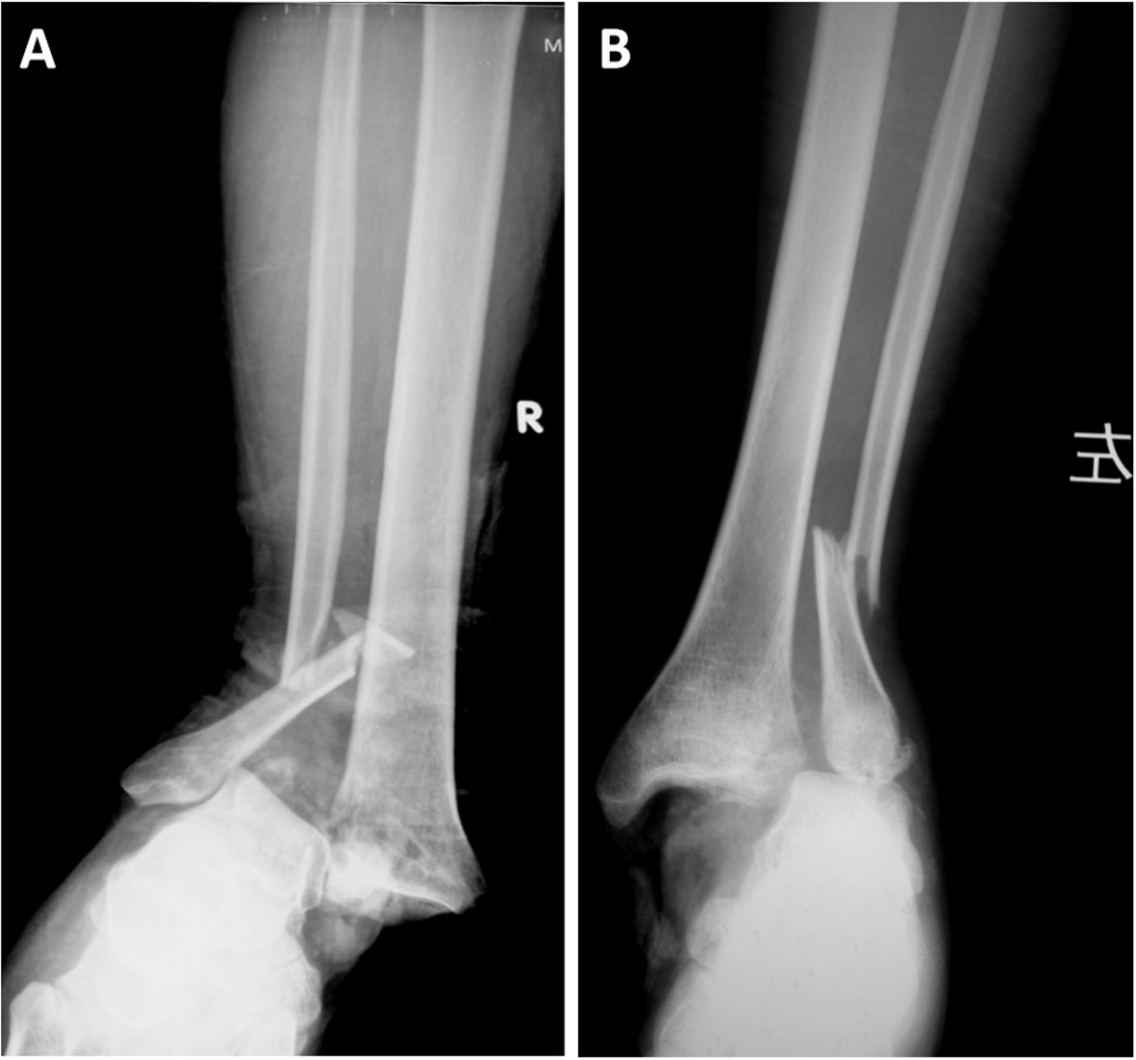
Characteristics of medial injury in patients with open Logsplitter injury of the ankle joint. A An avulsion fracture of the medial malleolus and talofibular joint congruence by anteroposterior X-ray film of the right ankle. B Deltoid ligament rupture and dislocation of the talofibular joint by anteroposterior X-ray film of the left ankle joint
